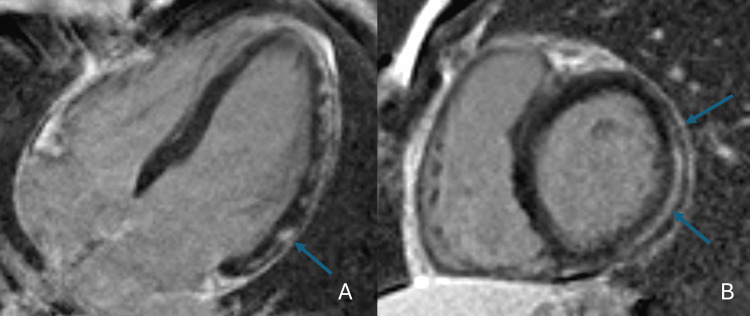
Cardiac MRI T1 PSIR images (A) Post-contrast image in the four-chamber view reveals patchy diffuse subepicardial and mid-myocardial late gadolinium hyperenhancement in the entire lateral wall (arrow). (B) Corresponding short-axis view reveals subepicardial to mid-myocardial hyperenhancement in the lateral wall and adjacent pericardium with minimal pericardial effusion (arrows). Findings are compatible with acute myocarditis and pericarditis. PSIR, phase-sensitive inversion recovery
